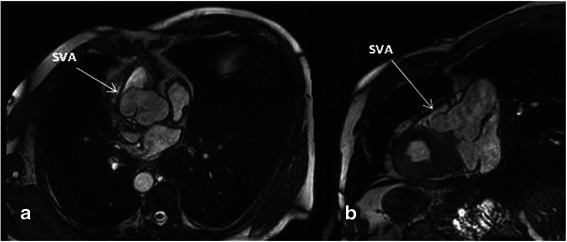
MRI: showing the right coronary sinus aneurysm in the axial (a) and sagittal plane (b)
